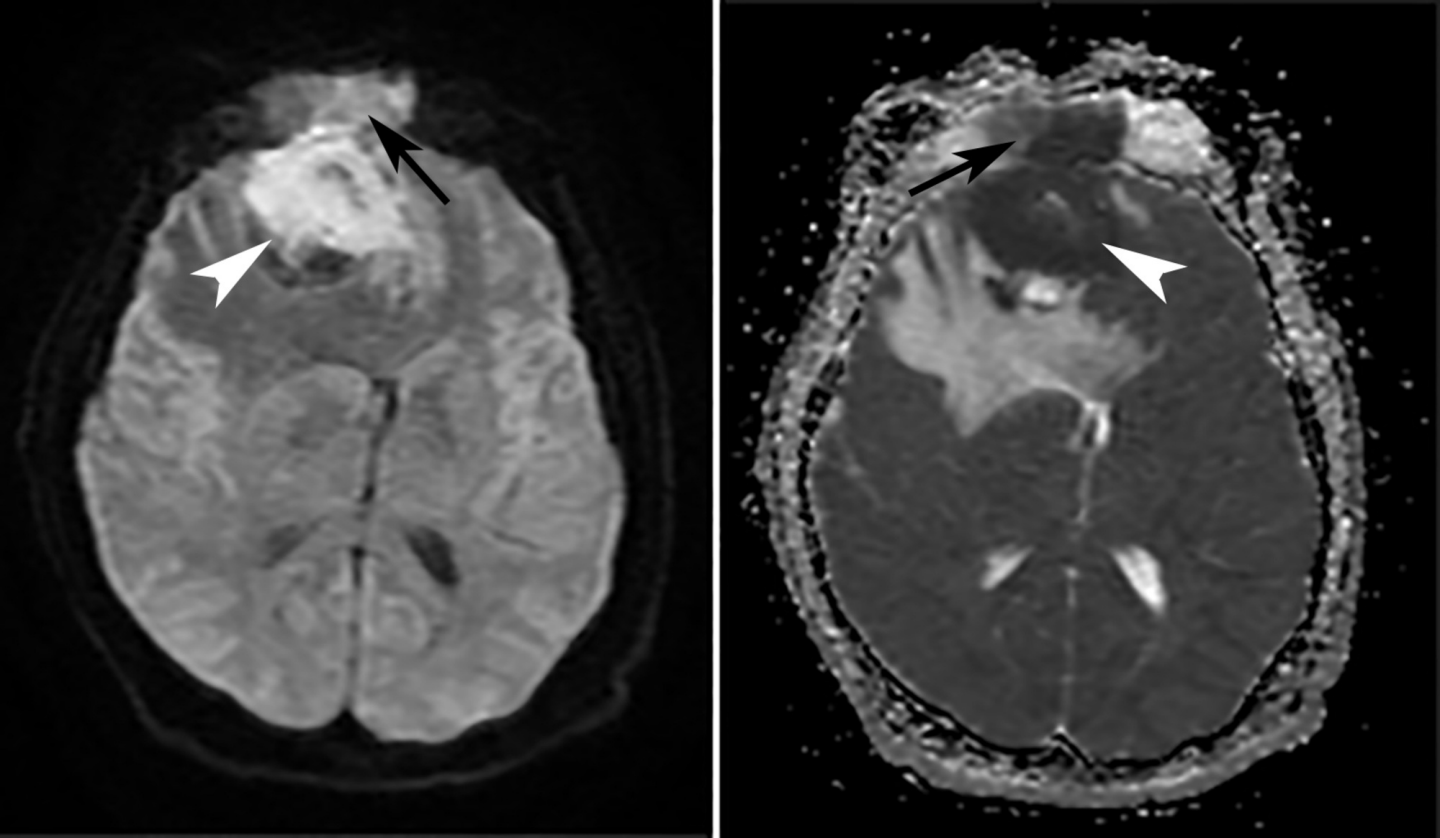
MRI head, DWI, and ADC Axial DWI (*left*) and ADC map (*right*) demonstrate an area of restricted diffusion (dark on DWI, bright on ADC) in a mass (*arrowheads*) involving the medial right frontal lobe and right frontal sinus (*arrows*), a finding suggestive of high cellularity. DWI: diffusion-weighted imaging, ADC: apparent diffusion coefficient
